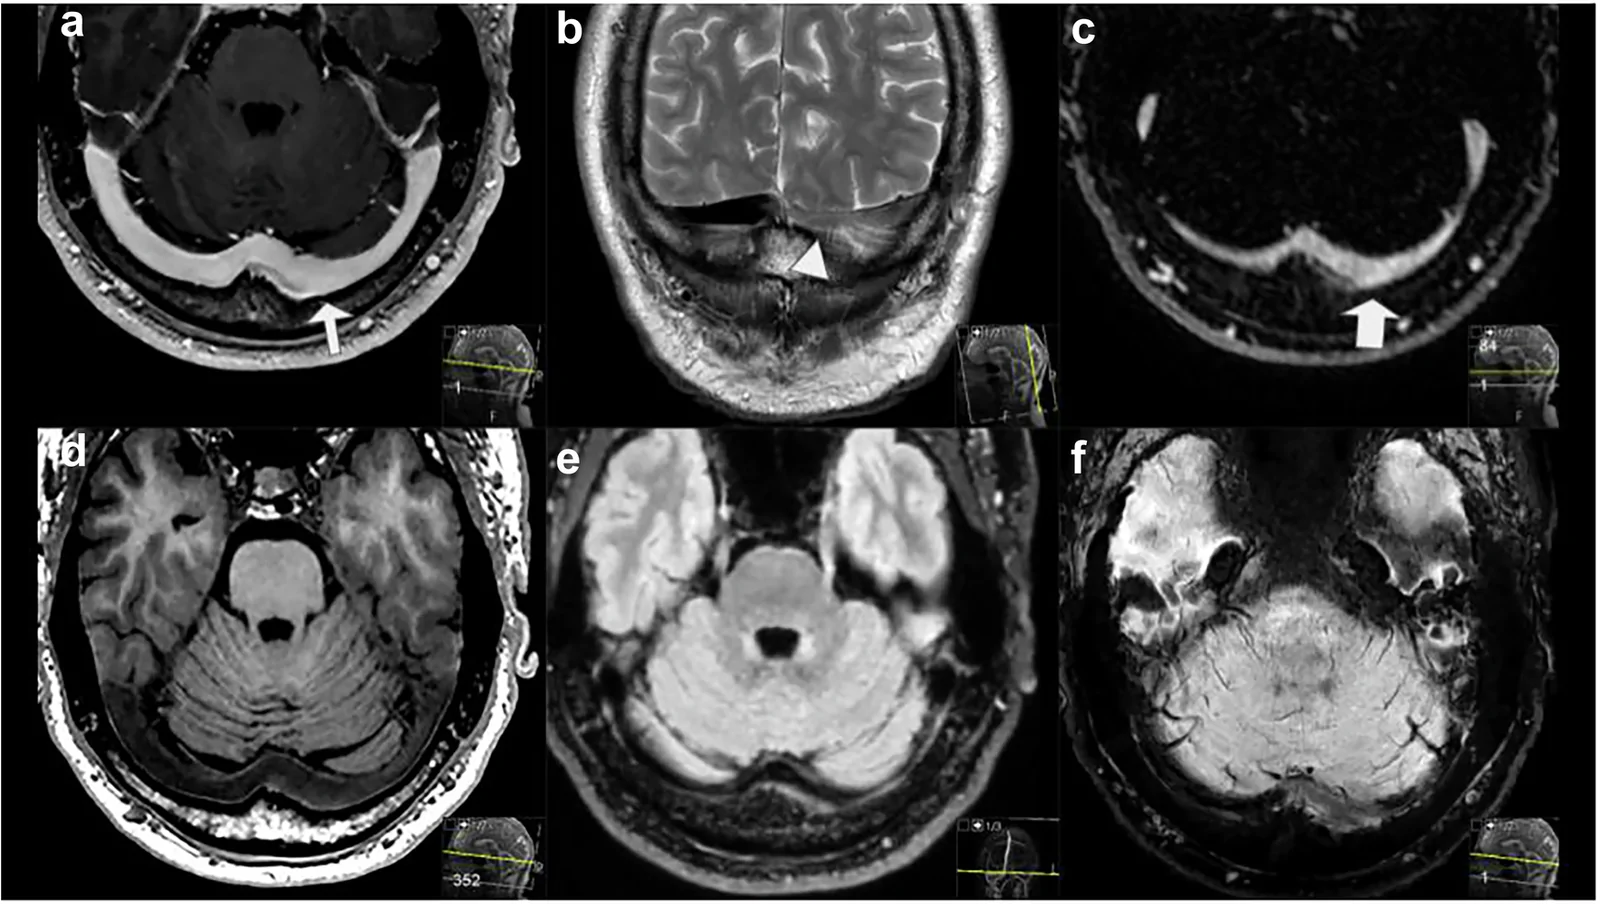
Follow-up excluding thrombosis. Upper row: a Post-contrast T1 showing total opacification (arrow), (b) T2 coronal with light signal changes (arrowhead), (c) time-resolved contrast-enhanced venography showing complete left transverse sinus opacification (fat arrow). Lower row: (d) T1, e T2-FLAIR, (f) SWI without signs of thrombus. Time-resolved MR venography confirms normal venous filling, ruling out thrombosis
